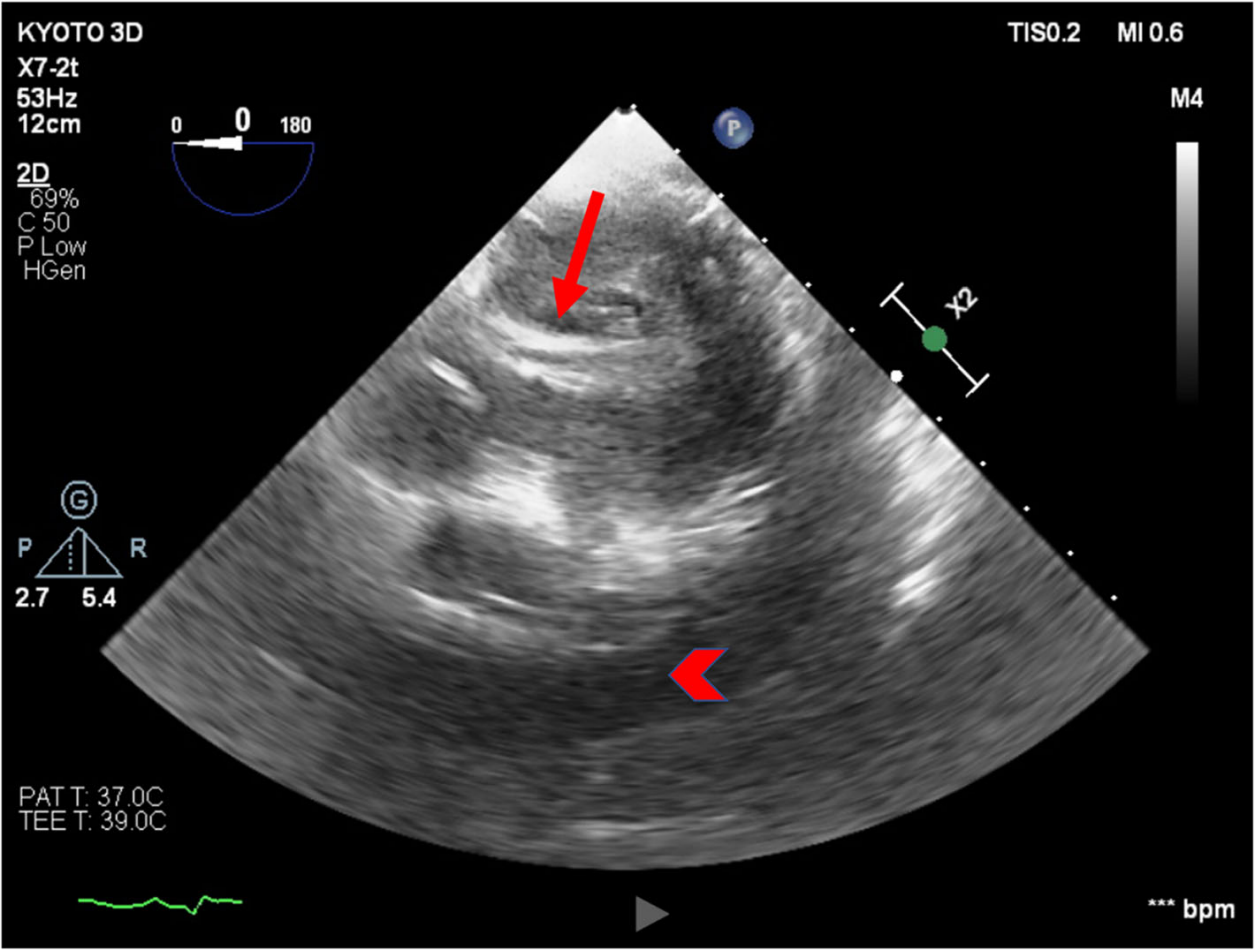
Transgastric apical short-axis view by transesophageal echocardiography. Note the massive pericardial effusion (arrow head) and, the part of the IMPELLA® (small arrow)
